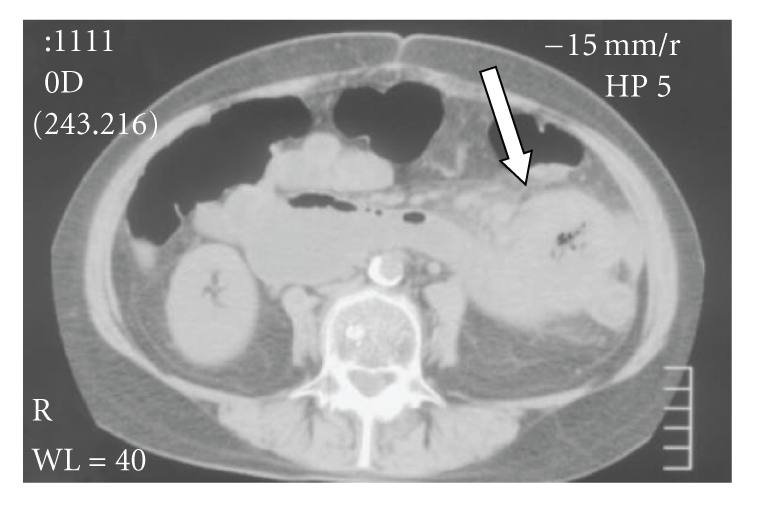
CT of the abdomen showing a solitary circular mass in the small bowel with surrounding fat infiltration.
